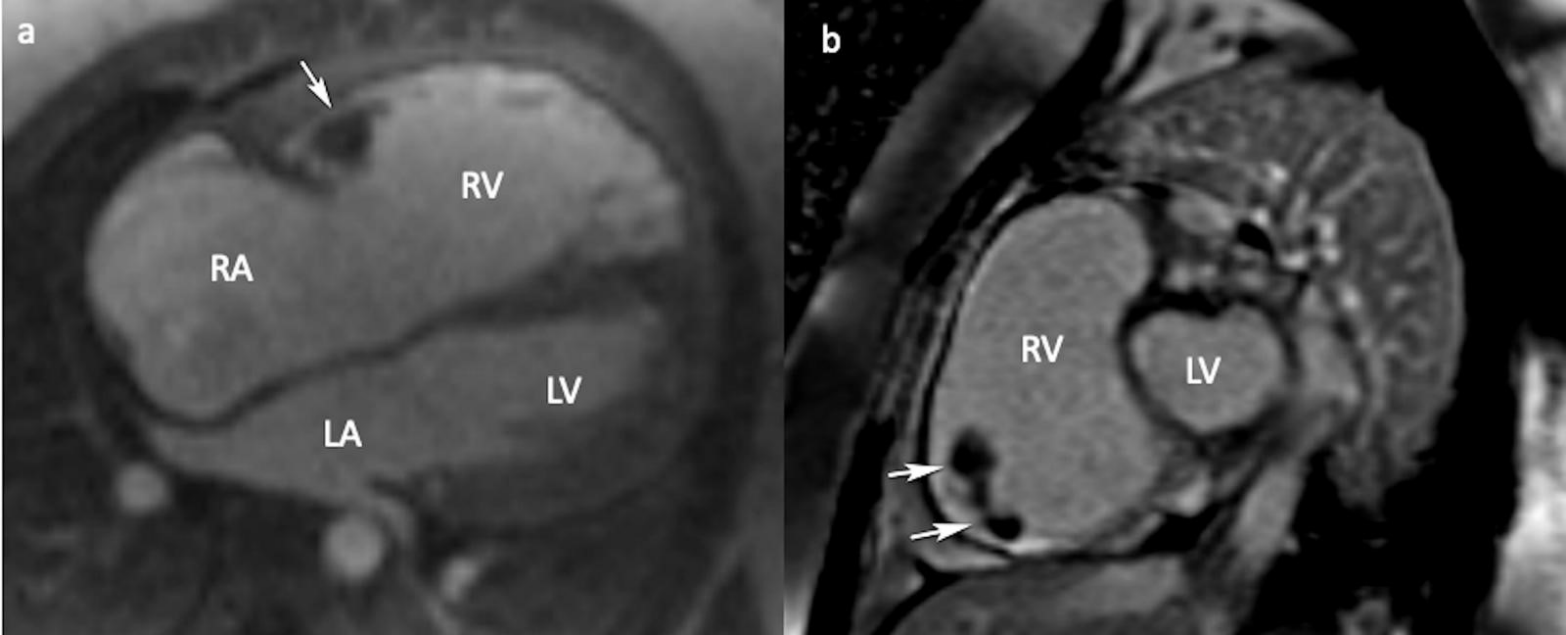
Concerning the RV thrombus, we initiated warfarin therapy and monitored the INR levels closely to ensure its efficacy. After achieving the targeted INR, a resolution of the thrombus-like mass was observed in the follow-up echocardiography after 3 months (Supplementary file 2, Video 2). ICD is contraindicated in situations where there are reversible causes (like myocardial ischemia, sepsis, hypoxia, electrolyte imbalance, electrocution, etc.) leading to VT/VF. For this reason, an ICD implantation was performed in our patient after the thrombus-like mass was resolved following medical treatment. An implantable cardioverter defibrillator (ICD) was implanted to the patient for primary prevention. The patient remains asymptomatic during follow-up visits and diligently attends scheduled appointments. Furthermore, proactive measures have been taken by inviting the patient’s first-degree relatives for cardiac disease screening.
